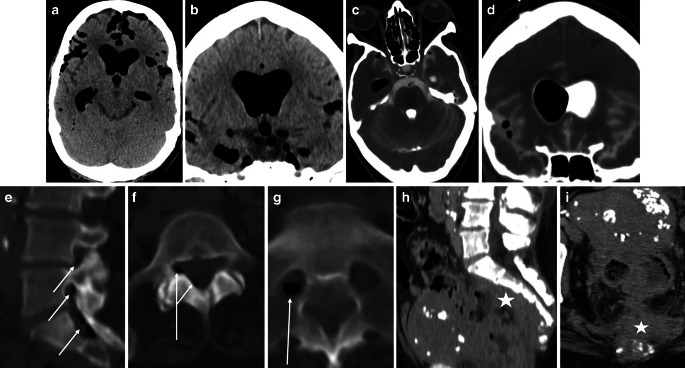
a, b Plain cranial CT scans of the ubiquitous air in the subarachnoid space and the ventricular system: a axial view, b coronal view. c, d Contrast-enhanced cisternography: c axial view, d coronal view. e–g Plain CT scan of the intradural lumbosacral pneumorrhachis (*white arrows*): e sagittal view, f axial view L5, g axial view S1. h, i Contrast-enhanced abdominal CT of the tumor and abscess in the retroperitoneal cavity and anterior sacral surface (*white star*): h sagittal view, i axial view
